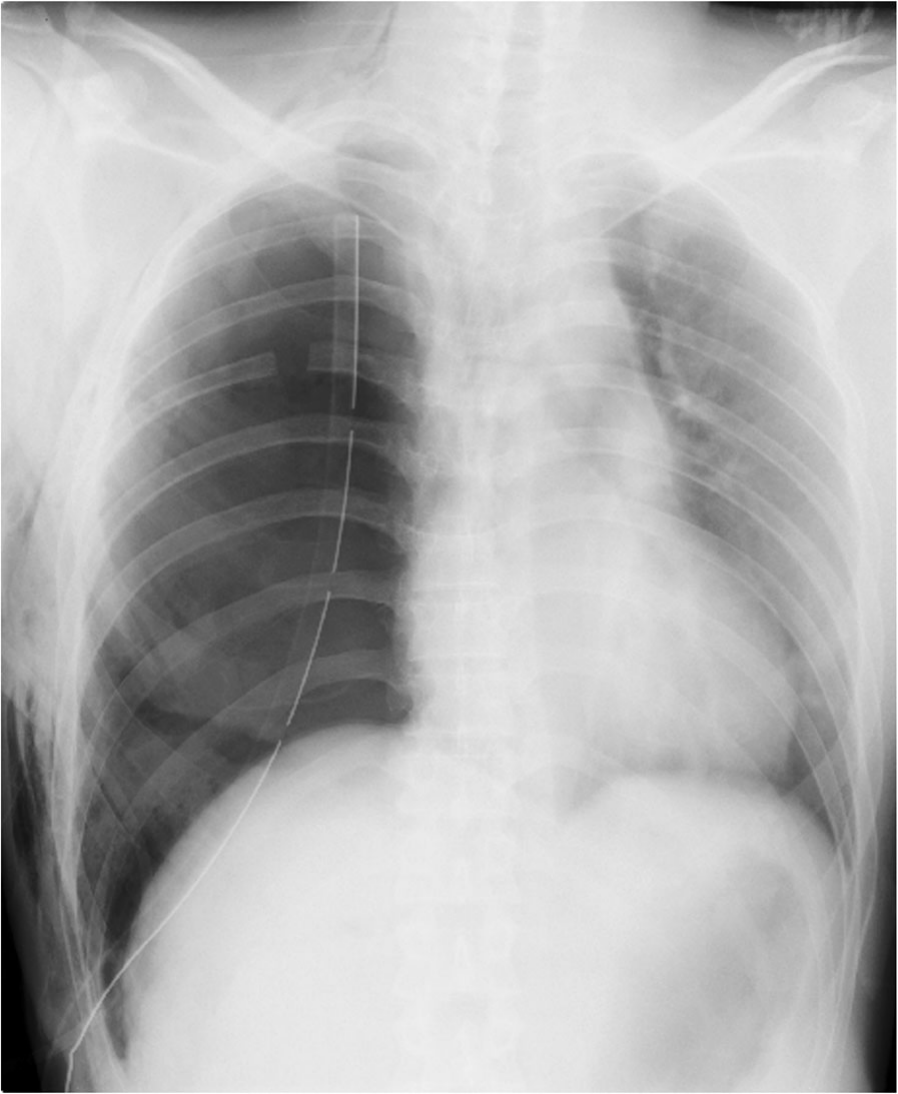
Chest radiograph before the elevation of the chest drain’s suction pressure from 7 to 15 cmH_2_O. This reveals mediastinal shift that may have been attributable to tension pneumothorax
